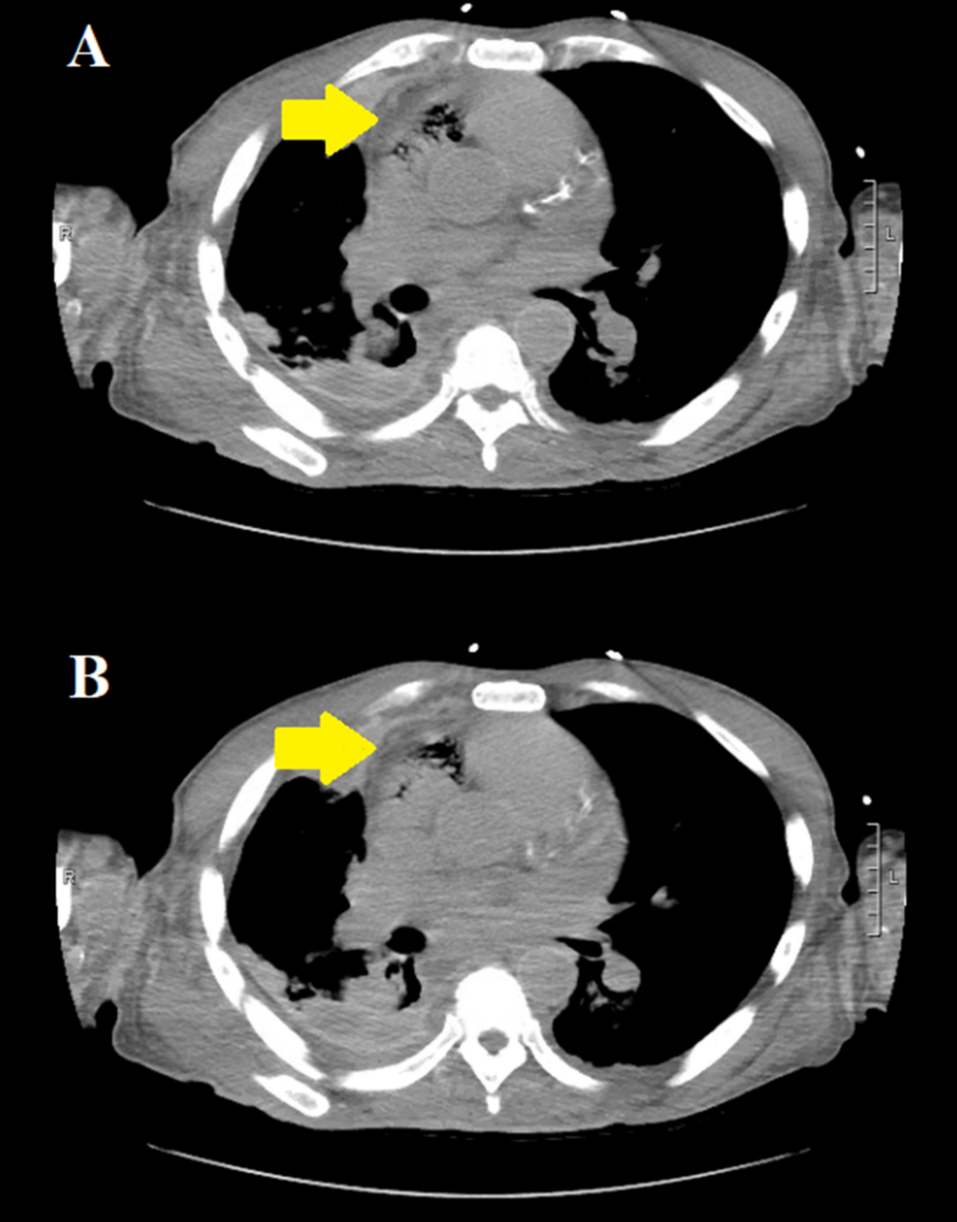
Repeat axial chest CT without intravenous contrast, 24 hours after the initial scan, demonstrating the same gas findings in the right atrium (yellow arrows in panes A and B); however of slightly less volume compared to the previous study.
